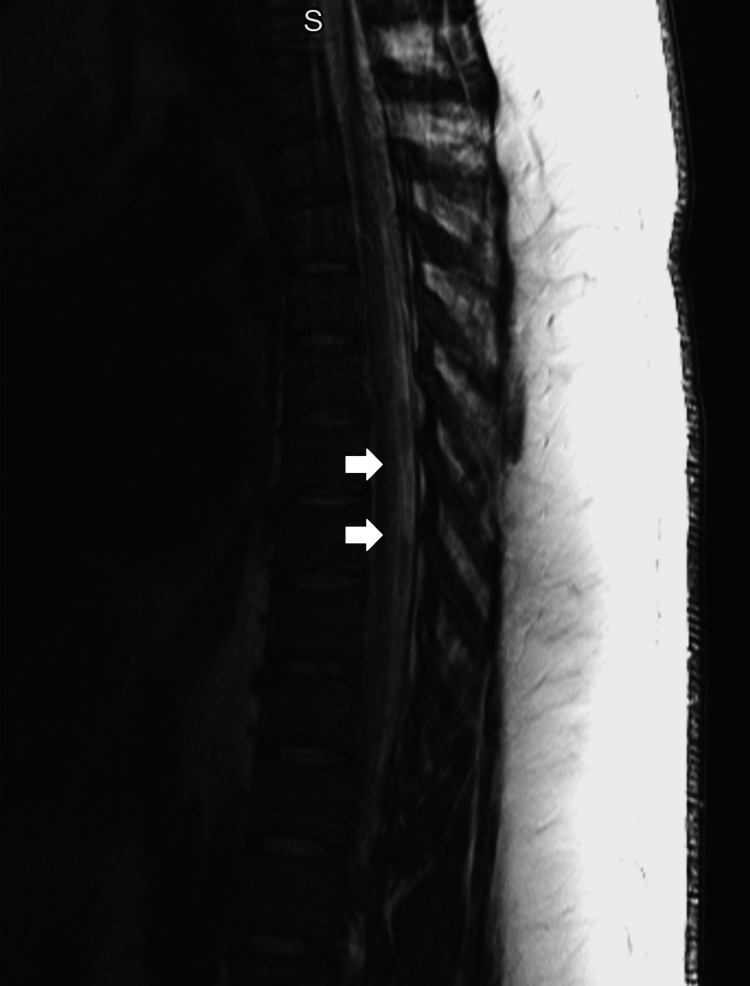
T2-weighted MRI of the thoracic spine (T-spine) showed diffuse central and posterior thoracic spinal cord signal abnormality, delineated by arrow tips, terminating near the lower end of the T-spine. A minor overlying artifact is present due to body habitus and motion near the superior T-spine.
